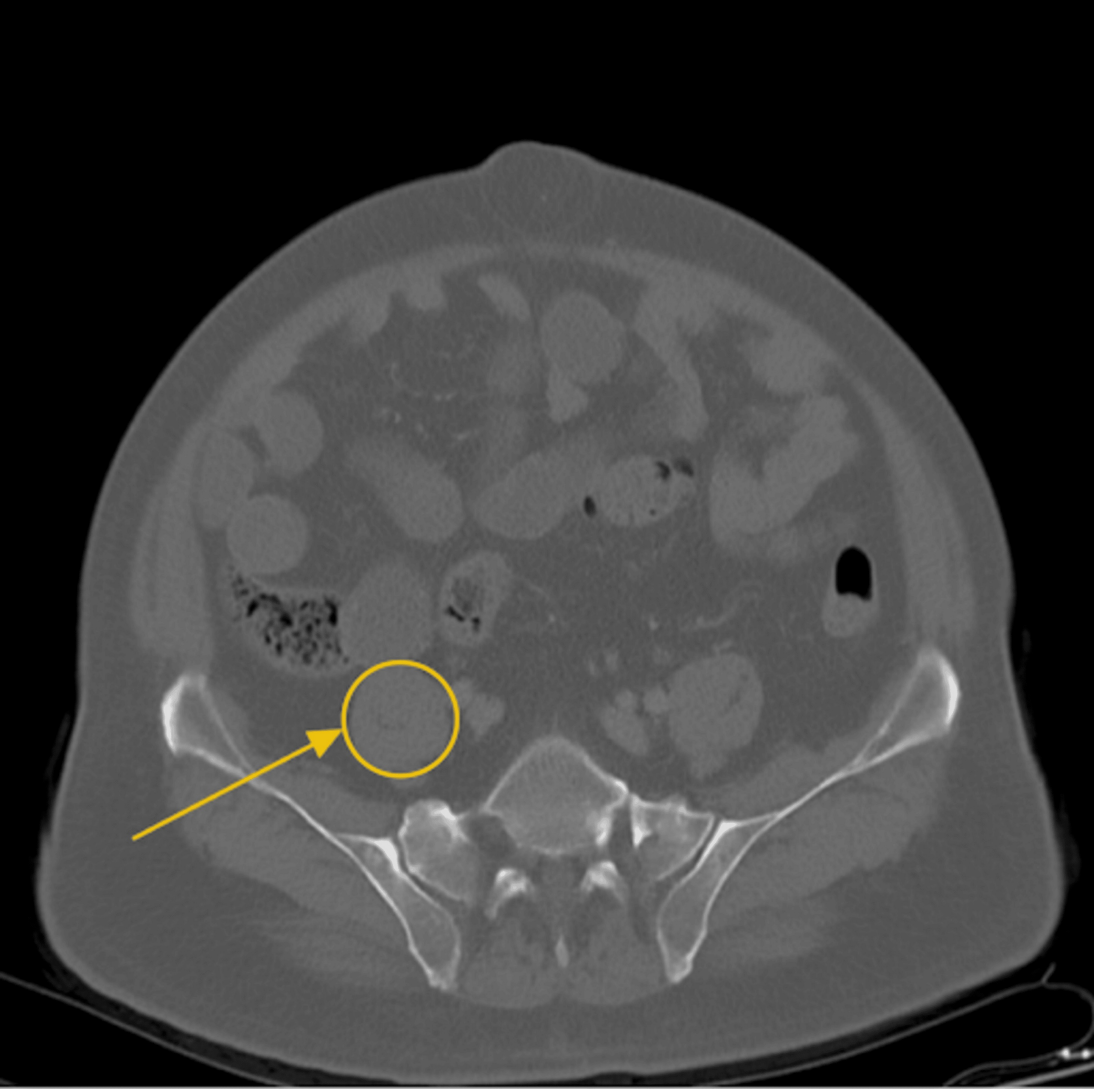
AM seen attached to the cecum on CT with contrast (transverse view) Dense tissue at the end of the cecum (yellow arrow and circle) indicates the incidental mass. AM, appendiceal mucocele
